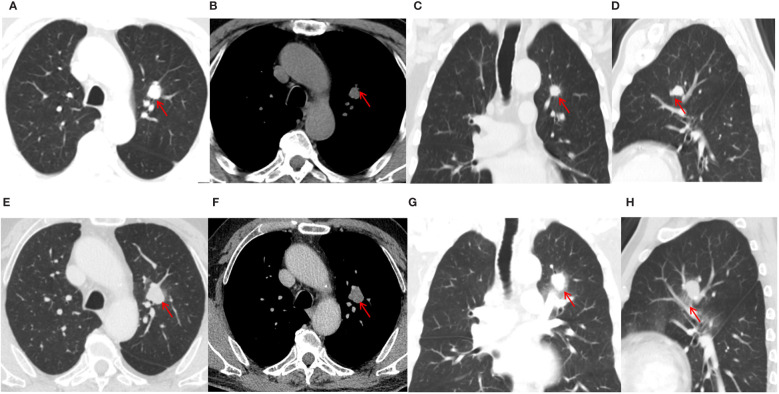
Chest CT of the patient. Plain CT on November 18, 2021 (A–D) and contrast enhanced CT on November 28 (E–H). Patches of ground glass opacity can be observed around the lesion on image (G, H).
